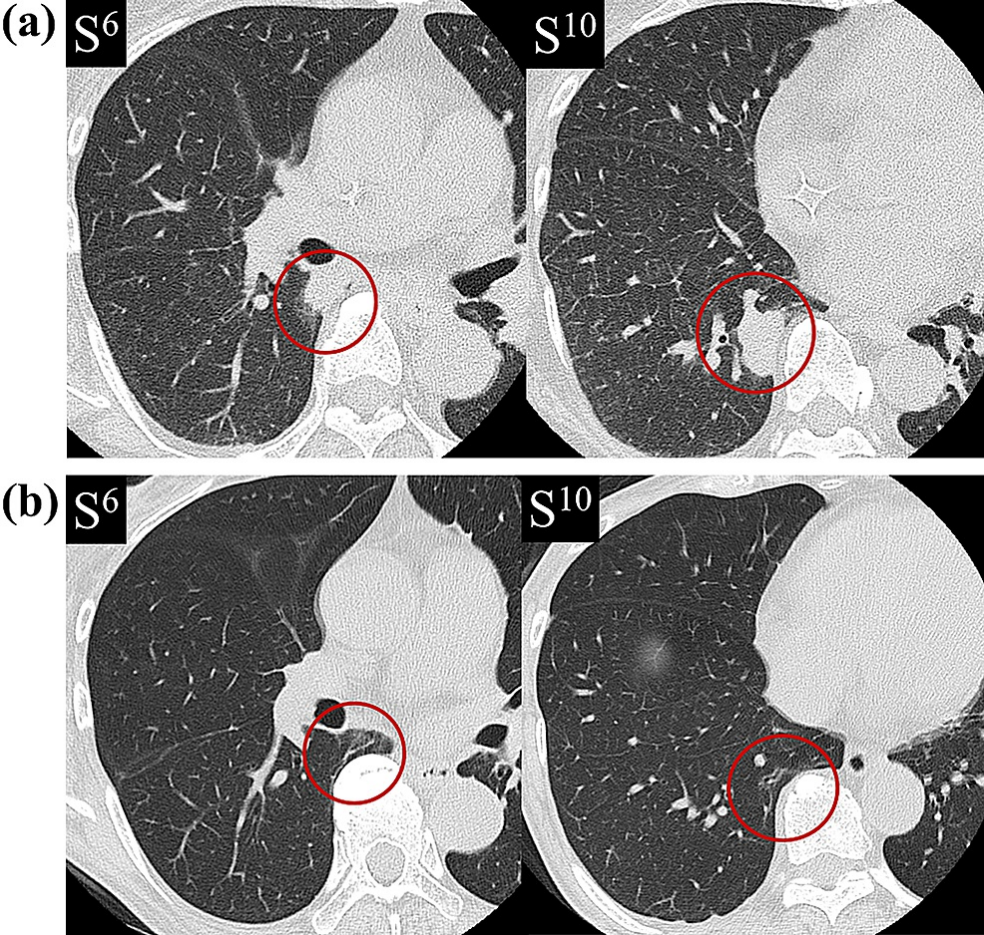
Chest CT before and after treatment. (a) Nine days after the previous CT examination, the masses became enlarged (20×13 mm and 28×19 mm). (b) CT showed the disappearance of the masses four months after starting treatment.
